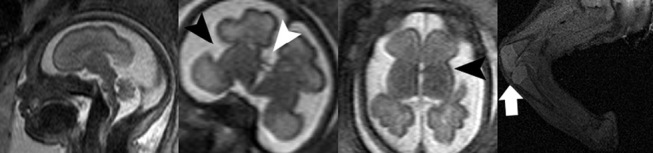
Fetal MRI in a case of genitopatellar syndrome at 32GW: note the delayed opercularization (black arrowhead, usually the insula should be covered by the adjacent frontal and temporal lobes), the absence of the corpus callosum (white arrowhead) as well as the simplified gyral pattern. The pathognomonic absence of the patella can be seen by fetal MRI as well, however is frequently missed – due to subtlety of this finding. In this case absence of the patella was diagnosed postnatally (white arrow).
